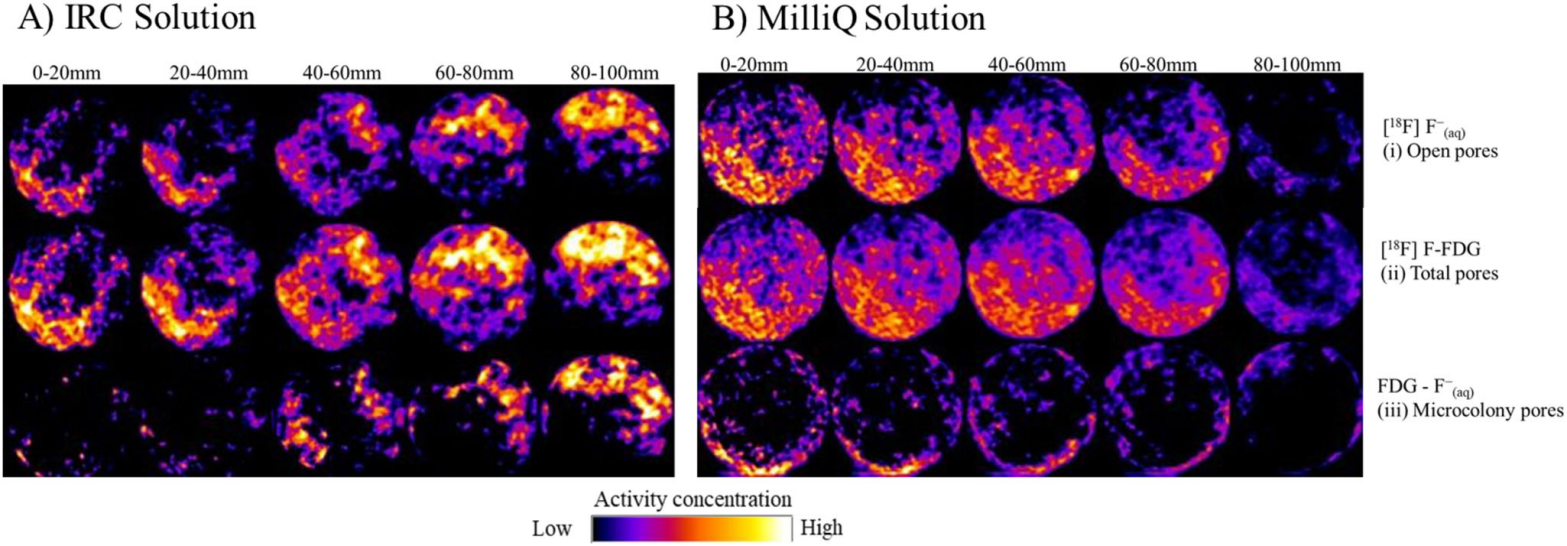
Mean radioactivity at different depths in columns packed with pea gravel and treated with (A) IRC solution and (B) Milli‐Q solution using positron emitting tomography. Radioactivity concentrations were adjusted relative to their maximum values to enhance visual comparisons between the images. Notably, the actual concentrations in the residual images were lower than the standardized maximum values. We differentiated between total pore volume as pores in which all the [^18^F]‐2‐deoxy‐2‐[^18^F]fluoro‐D‐glucose (FDG) was present (ii) and open pores in which only [^18^F]Fluoride (F)^−^
